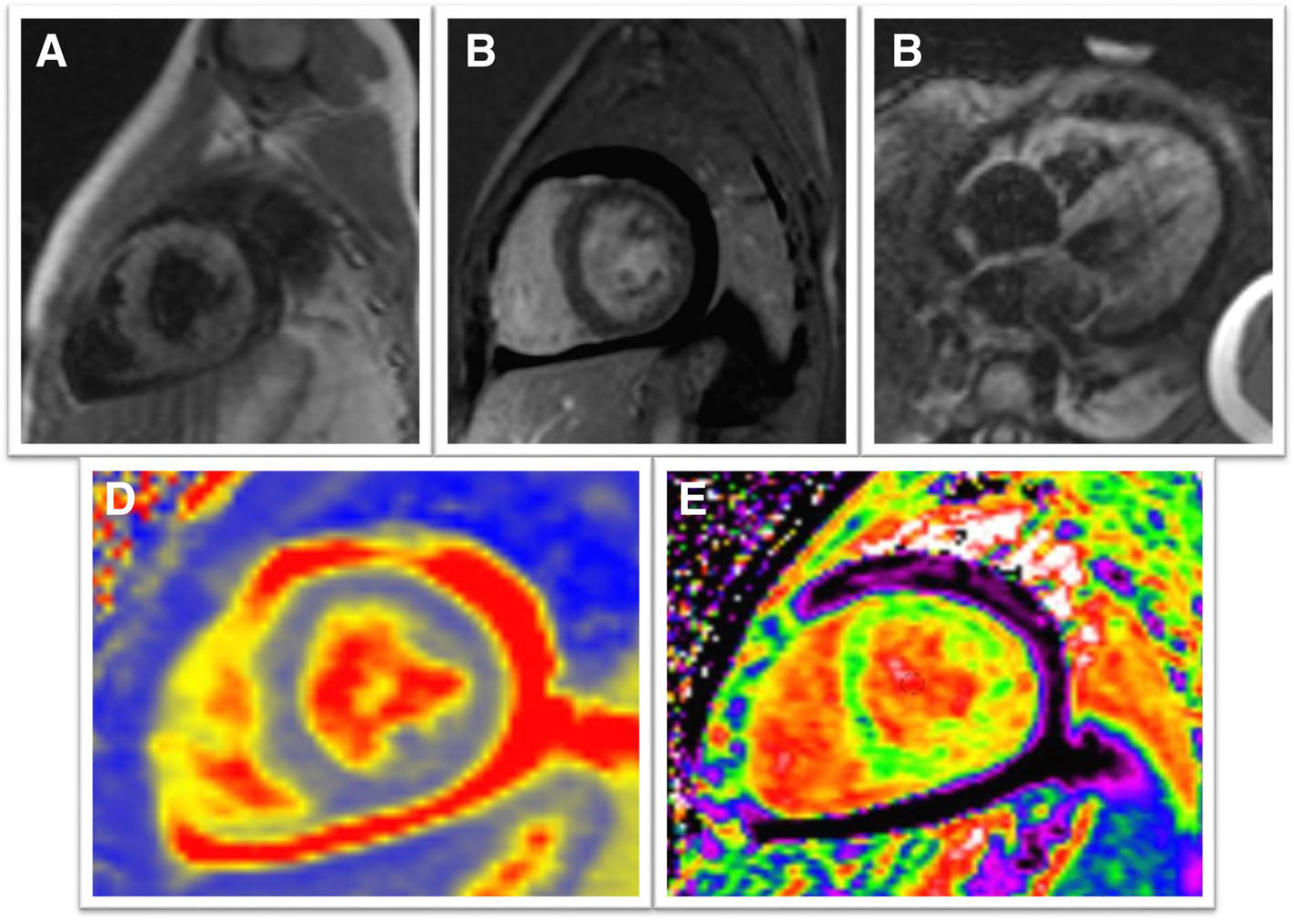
Selected cardiovascular magnetic resonance (CMR) images from a 1.5 year old girl with a clinical diagnosis of acute myocarditis. The top panel demonstrates normal selected images from the Lake Louise criteria (LLC), with: (a) no increased T2-weighted (T2W) signal intensity, (b) no evidence of late gadolinium enhancement (LGE), (c) no increased early gadolinium enhancement (EGE) and a pericardial effusion. The bottom panel demonstrates abnormal (d) T2M and (e) ECV maps in the mid-portion of the short-axis. The global native T1 was 1270 ms, the global T2 was 60 ms and ECV was 39.6%
